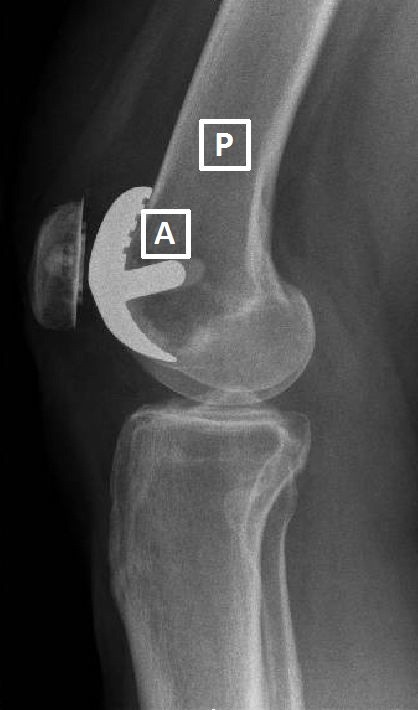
Location of anterior (A), and proximal (P) regions of interest (ROI) on a lateral radiograph of a right knee following Richards II patellofemoral joint replacement.
